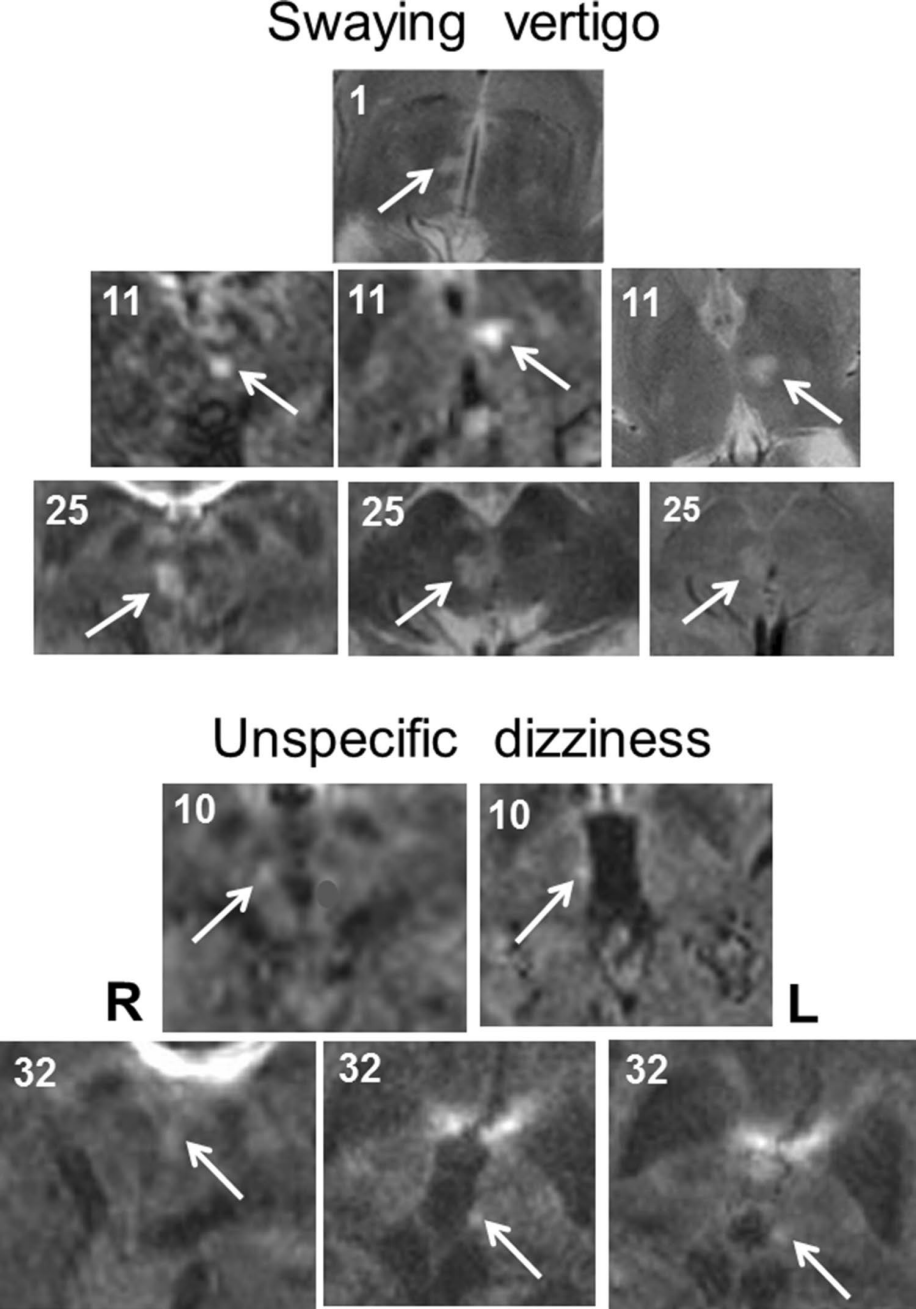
Transverse sections of MRI scans of five patients (nos. 1, 10, 11, 25, and 32) who manifested with acute swaying vertigo (upper panel: nos. 1, 11, and 25) or unspecific dizziness (lower panels: nos. 10 and 32) (diffusion- and T2-weighted sequences). In these patients, the unilateral midbrain strokes affected more rostral structures including the oculomotor nucleus, the interstitial nucleus of Cajal, and the rostral interstitial nucleus of the MLF (nos. 11 and 25) or meso-diencephalic structures up to the paramedian thalamus (nos. 1, 10, and 32), see also Table 2. *MLF* medial longitudinal fascicle
